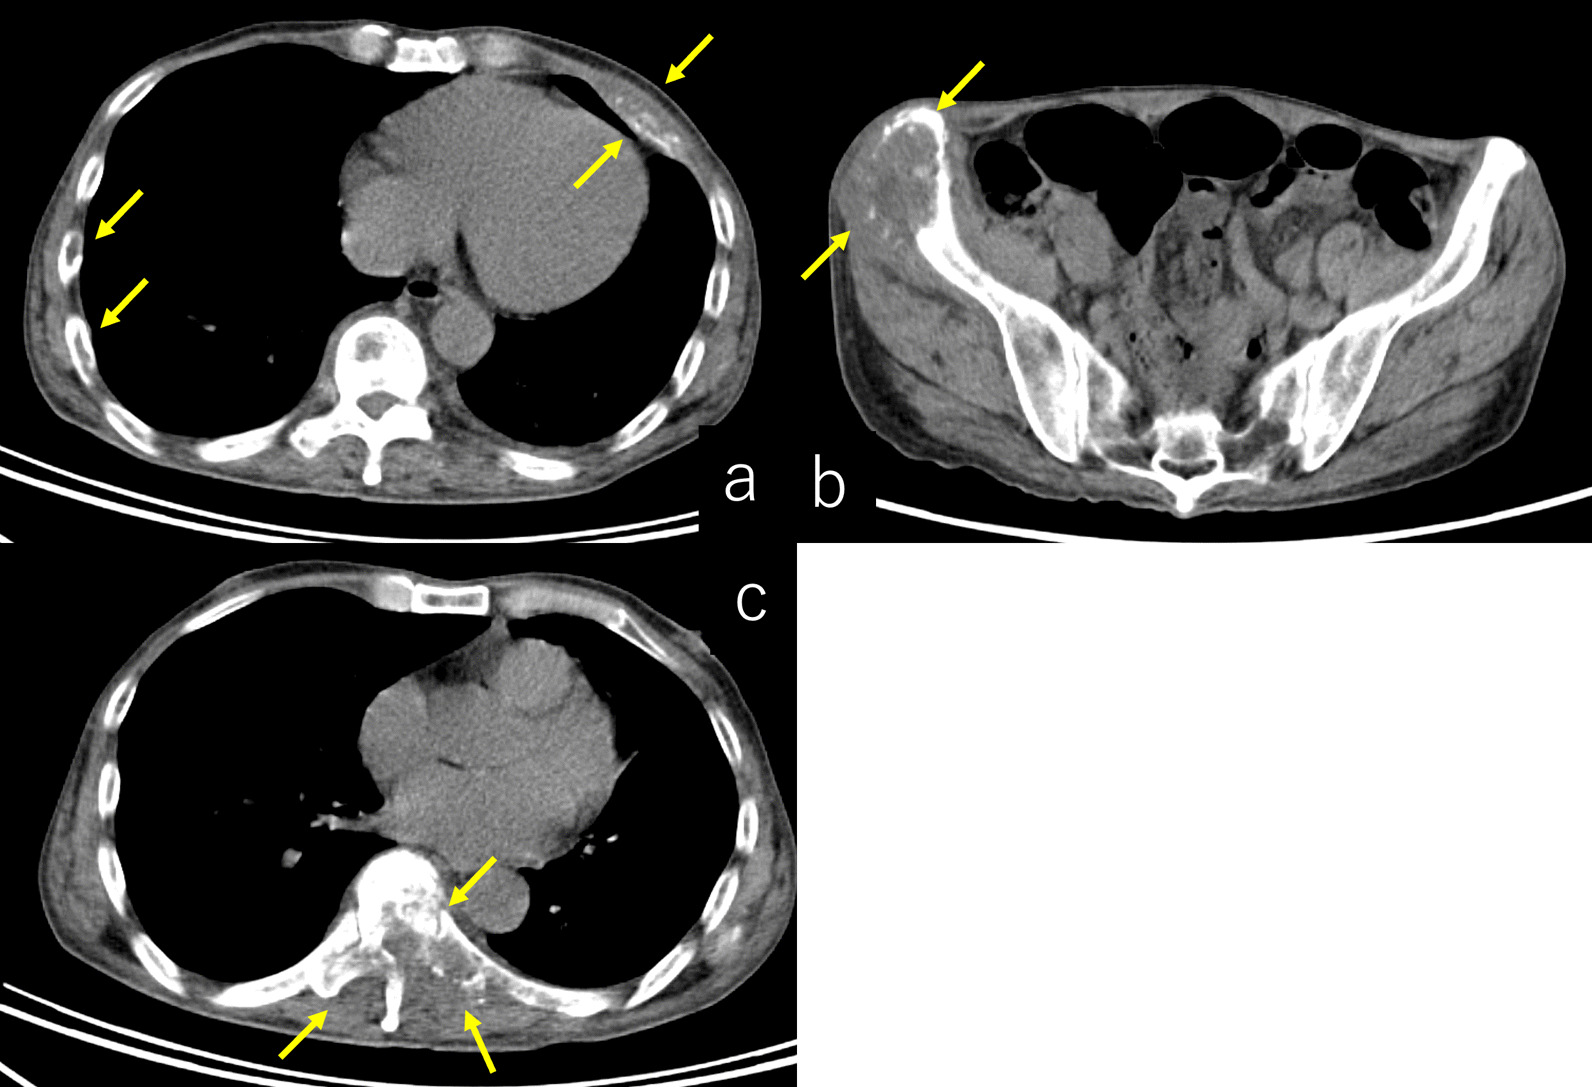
Computed tomography showed lesions in left eighth rib (a), right iliac bone (b), and ninth thoracic vertebra (c) (yellow arrows)
